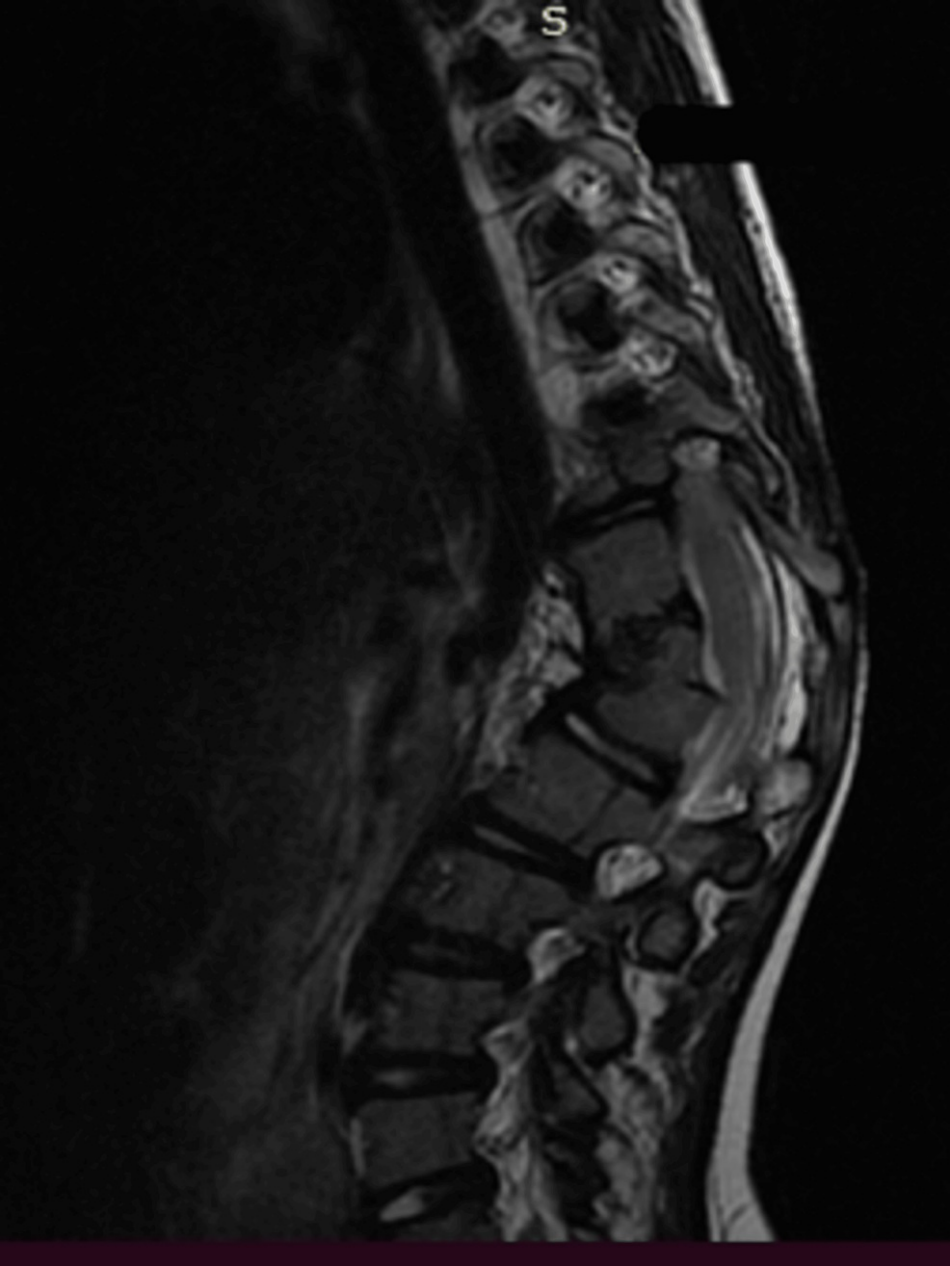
A pre-operative MRI of the thoracolumbar spine depicts the kyphosis level and the hemivertebrae.
